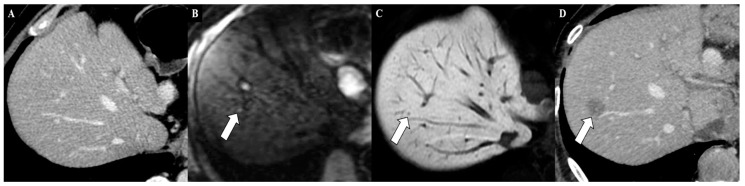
Axial contrast-enhanced computed tomography (CT) image (A), in the same patient, did not show metastatic lesions at this liver level. The axial gadoxetic acid-enhanced magnetic resonance imaging, performed using diffusion-weighted imaging (B) and during the hepatobiliary phase (C), demonstrated a small liver metastasis in the liver segment VIII (white arrows). After six months, the axial contrast-enhanced CT image (D) confirmed the metastatic liver lesion (white arrow), enlarged.
